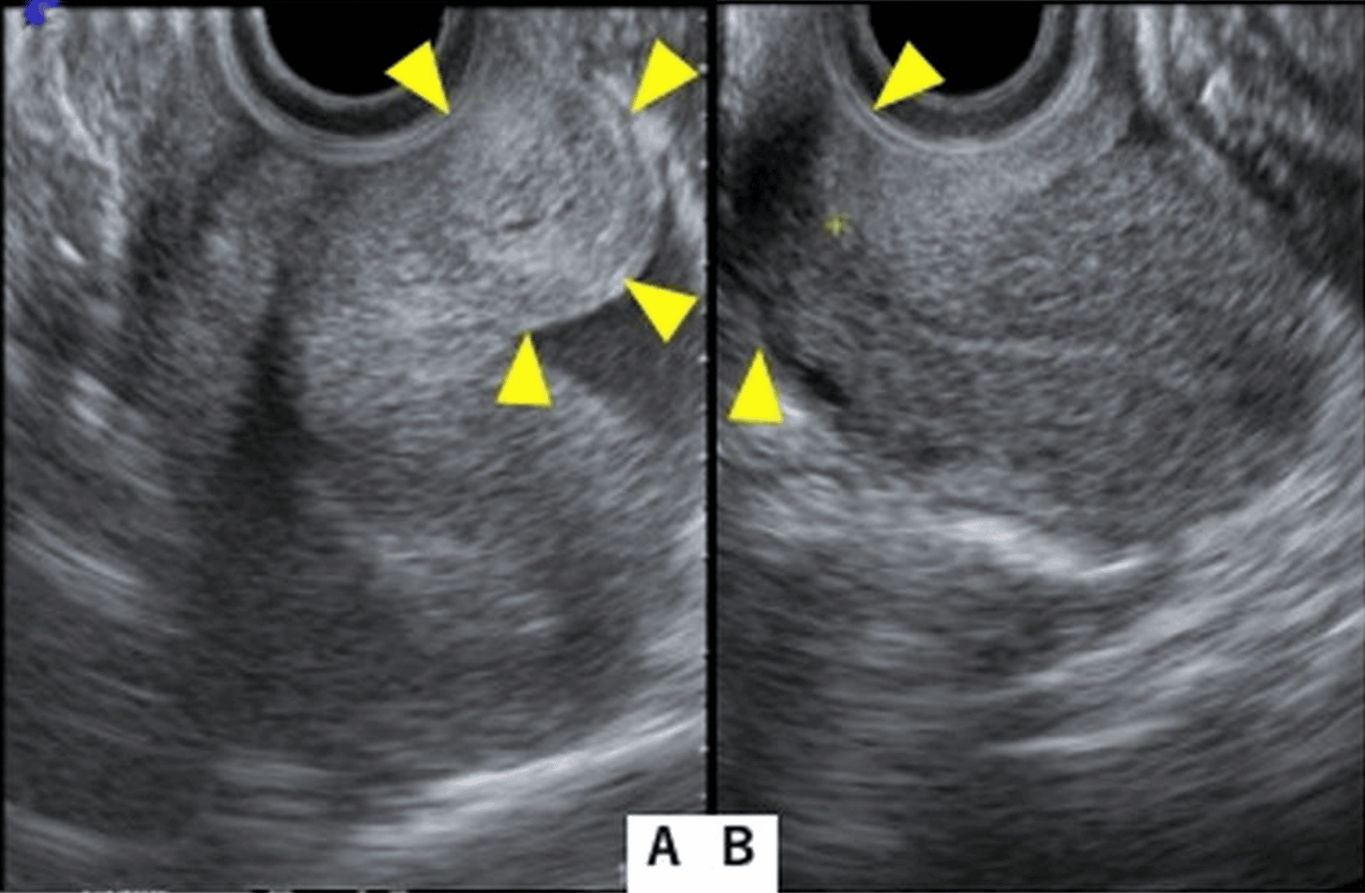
Ultrasonography findings. (A) Vaginal ultrasonography at the first visit shows swelling of the cervix (yellow arrowheads). (B) Vaginal ultrasonography performed after three months shows normal findings of the cervix (yellow arrowheads).
